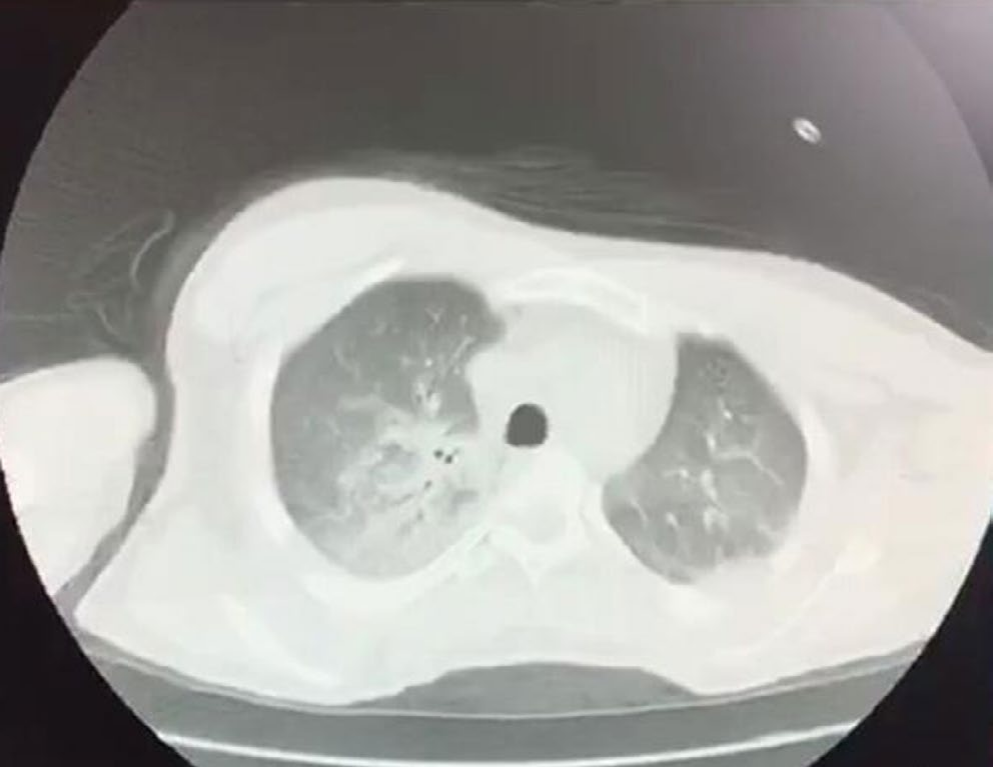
Chest CT scan showing bilateral pulmonary infiltration suggestive of COVID‐19 pneumonia
